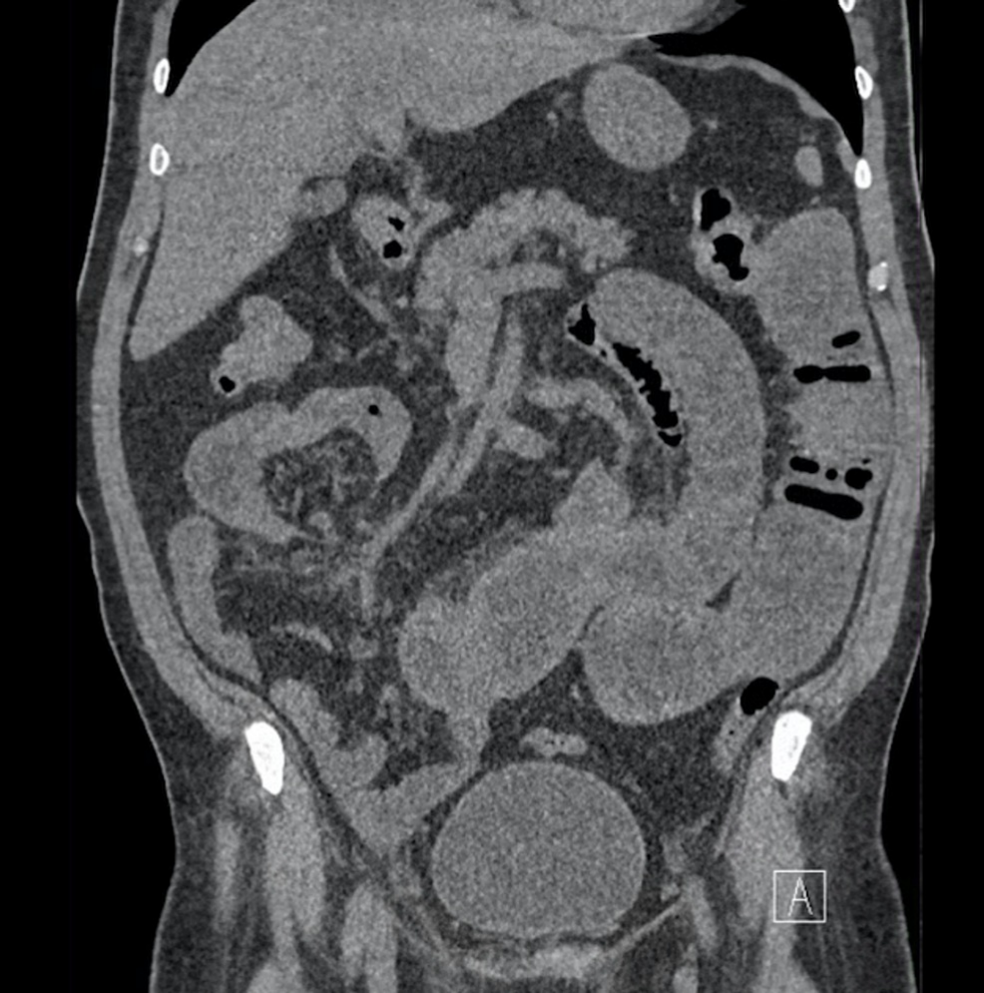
Coronal non-contrast CT KUB image CT: computed tomography; KUB: kidneys, ureters, and bladder
